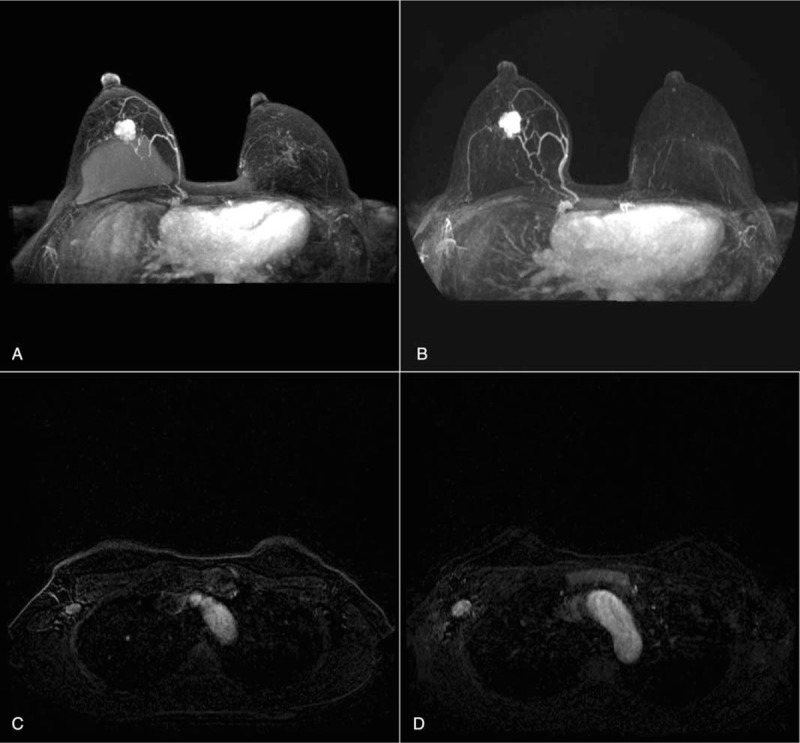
At the time of diagnosis, initial breast MRI was compared with those performed 3 times after chemotherapy. A, Initial breast MRI for breast tumor. B, Initial breast MRI for axillar metastatic lymph node. C, Breast tumor after chemotherapy. D, Axillar metastatic lymph node after chemotherapy. MRI = magnetic resonance imaging.
